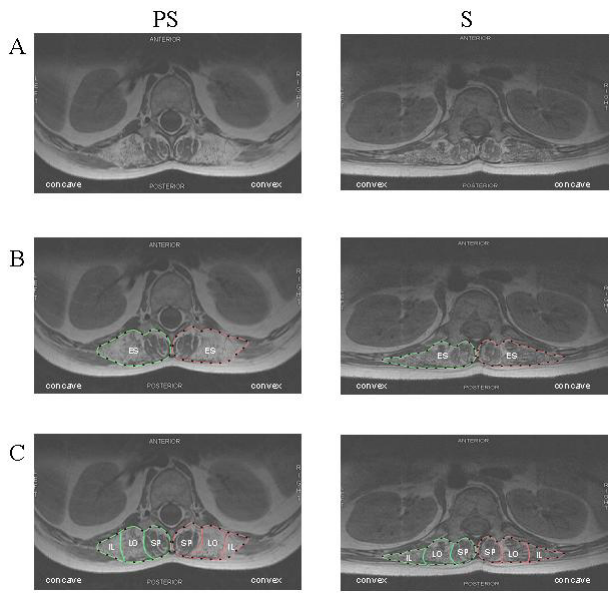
MR images obtained at L2 level for the pre-scoliotic (PS) patient P6 (left column) and for the scoliotic (S) patient P7 (right column). A: original images; B: manually obtained contours of the erector spinae (ES); C: manually obtained contours of the spinalis (SP), longissimus (LO), and iliocostalis (IL).
